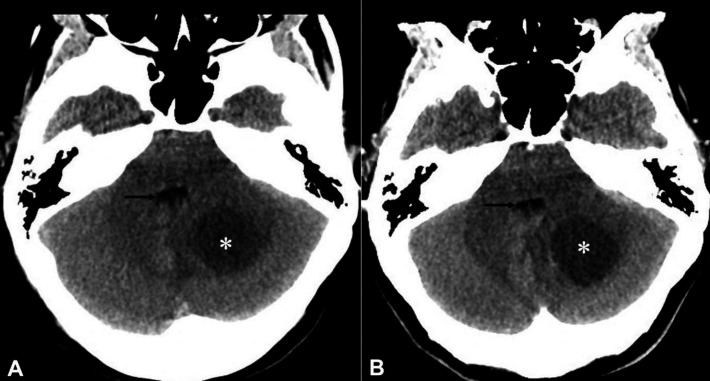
Non-contrast (A) and contrast enhanced (B) CT brain of a 24 year-old female with headache and increasing unsteadiness shows a hypodense area in the left cerebellar hemisphere (asterisk) with faint peripheral enhancement. Note the mass effect on the 4th ventricle (black arrow) by the mass. The main differential diagnosis of a low density cerebellar mass in a young adult is pilocytic astrocytoma. Other pathologies to occur in the posterior fossa of a young adult include hemangioblastoma, ependymoma, medulloblastoma, metastasis and lymphoma
